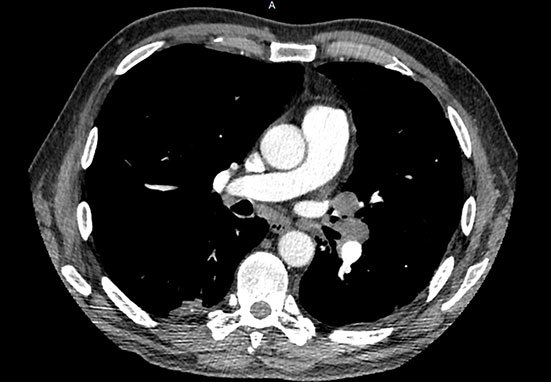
Axial CT image showing enlarged non-calcified hilar nodes and a partially calcified pleural-based pulmonary nodule.
